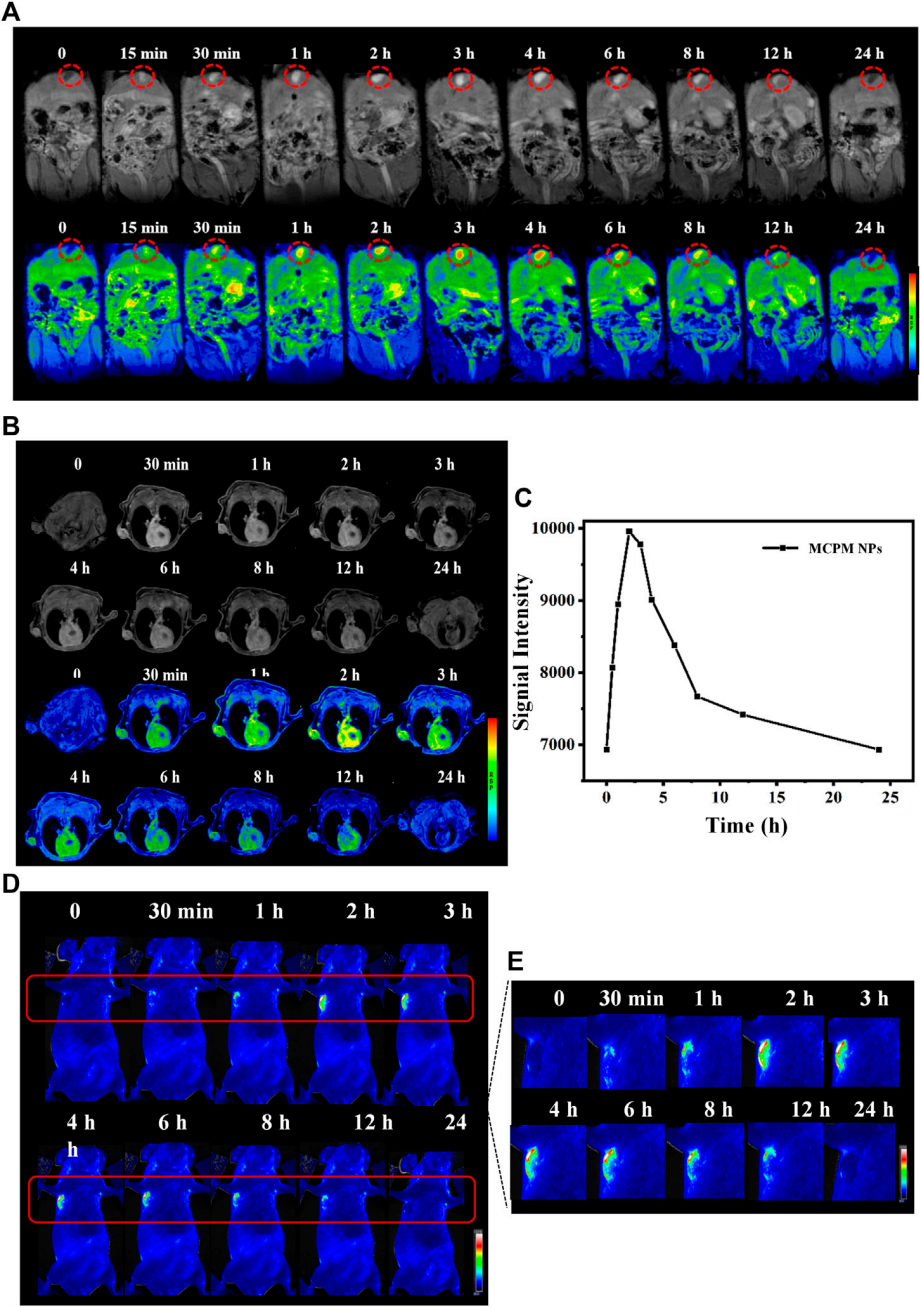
MRI/FL ability of MCPM NPs. (A)
